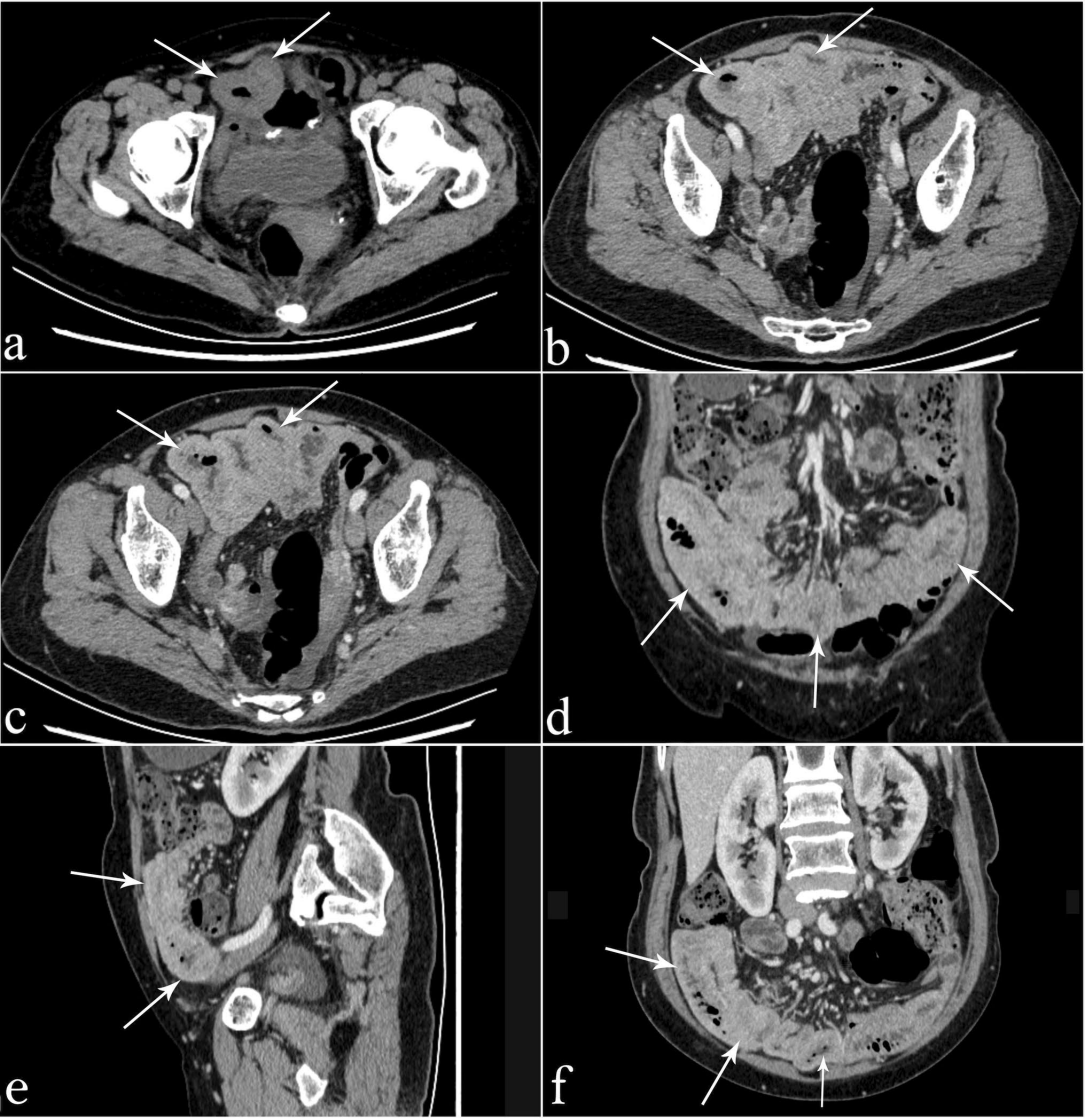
A 66-year-old female patient with adhesive SBO and SBN underwent radical surgery for sigmoid colon cancer 4 years ago, with symptoms of abdominal pain and vomiting. After conservative treatment outside the hospital, small-bowel dilation was slightly alleviated, but symptoms such as abdominal pain did not show significant relief. a. Thickened small-bowel wall can be seen in the lower abdomen, presenting as an overall high-density target sign (arrows) on CT plain scanning, and the CT value of the diseased small-bowel wall is approximately 41 HU. b and c After enhanced scanning, the thickened small-bowel wall with a high-density target sign shows an increase in enhancement. After enhancement, the CT value of the small-bowel wall is 93 HU with significant enhancement, and the CT value of the diseased small-bowel wall increases by approximately 52HU. d and e The coronal and sagittal views show an thickened small-bowel wall with the overall high-density target sign (arrows). f The arrow indicates an overall high-density target sign with significant enhancement in 3D MPR
